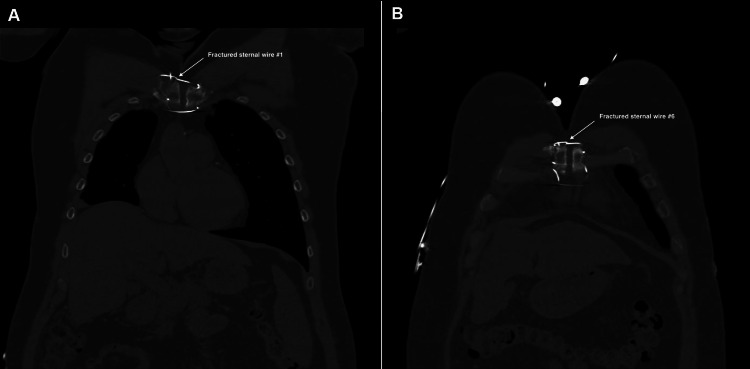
Preoperative CT imaging of the chest (coronal section) showing chronic sternal non-union as well as fractured first and sixth sternal wires The image shows a chronic non-union of the sternum, as well as fractured wire number 1 (panel A) and wire number 6 (panel B) CT: computed tomography
